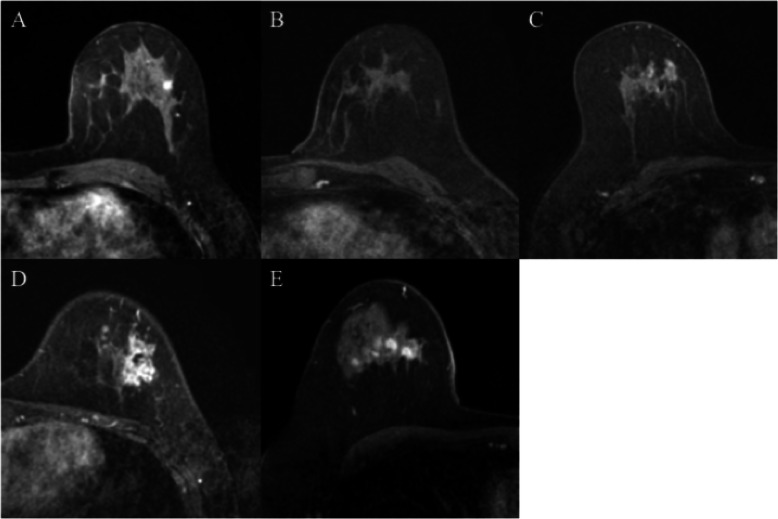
Magnetic resonance images of the shrinkage patterns of triple-negative breast cancer following neoadjuvant chemotherapy. a Concentric shrinkage without any surrounding lesions, type I; b non-visualization; c residual multinodular lesions, type III; d concentric shrinkage pattern with surrounding lesions, type II; e diffuse contrast enhancement in entire quadrant, type IV
